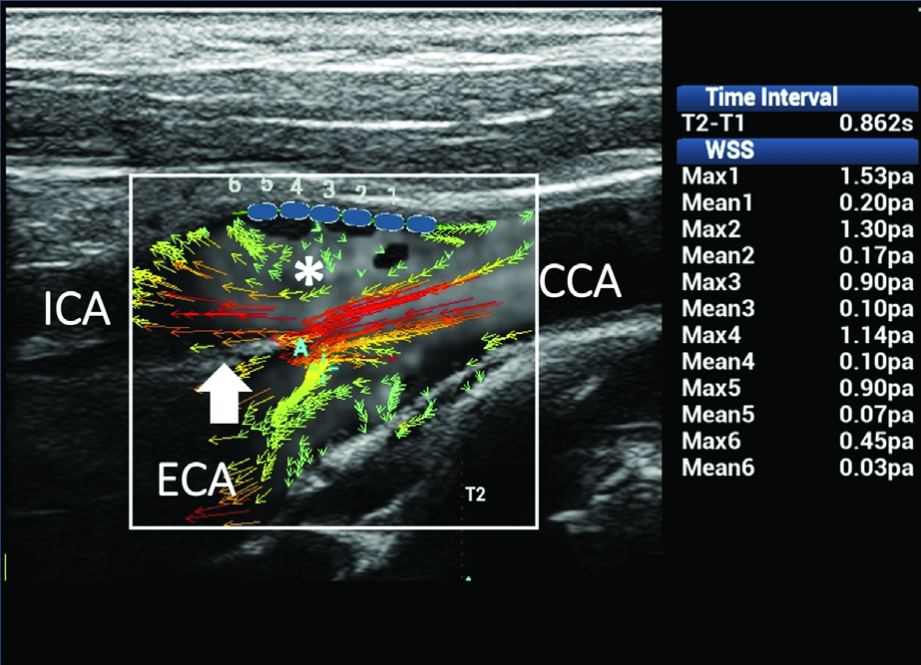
Reversed flow in the CB. Ultrasound vector flow imaging shows high‐velocity red vectors moving forward along the flow divider in the internal carotid artery (ICA; arrow). Some low‐velocity green vectors move back on the opposite wall to the flow divider in the ICA sinus (asterisk). Wall shear stress measurements (blue dots) at that level gave about normal maximum values (0.90–1.53 Pa) in 5 of the 6 samples and abnormal mean values (0.03–0.20 Pa) in all of the sample points as a consequence of the unsteady shear stress. CCA indicates common carotid artery; and ECA, external carotid artery.
